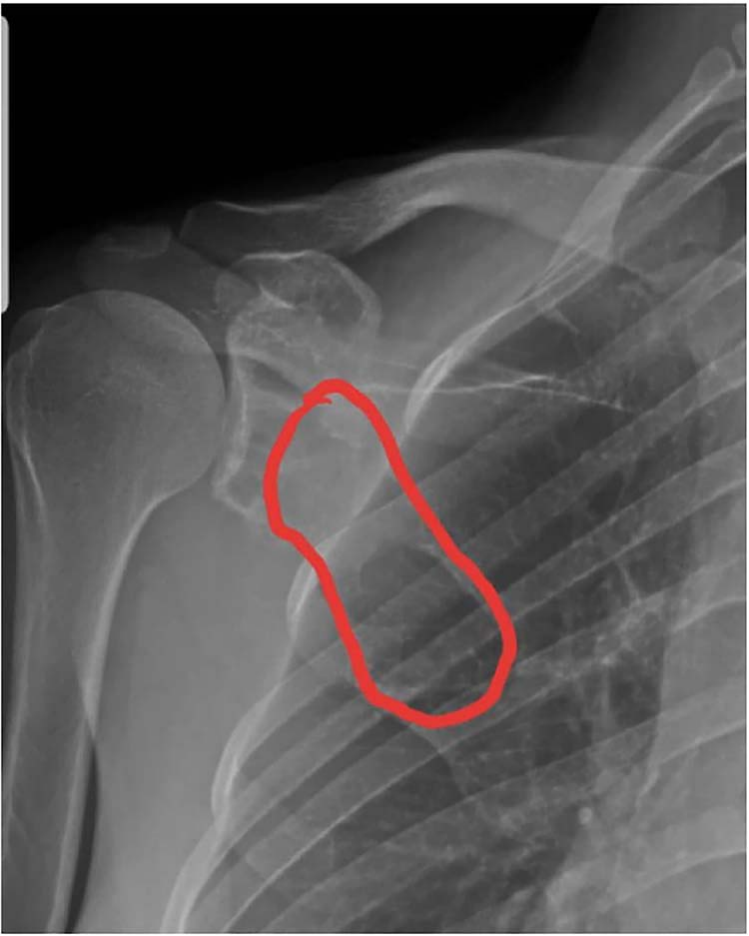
X-ray of the right shoulder showing an ill-defined, eccentric, lucent lesion in the right scapula.
